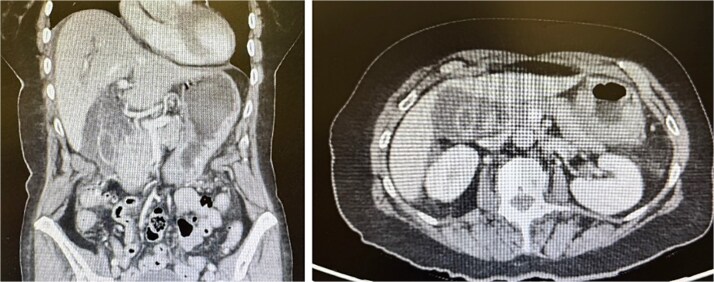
CT abdomen and pelvis with contrast. The gallbladder is severely thick walled with intramural oedema. Extensive pericholecystic soft tissue stranding is seen. The adjacent free fluid is noted extending into the subcapsular region. There are no biliary dilatation or gallstones. No concerning lesion is seen in the liver, pancreas, spleen, kidneys, or adrenal glands. No obvious hydronephrosis or renal tract calculus is seen. The bowel loops are not dilated and there is no bowel mass seen. There is no free gas or fluid seen. No abdominal or pelvic adenopathy is seen. Findings in keeping with acute cholecystitis.
